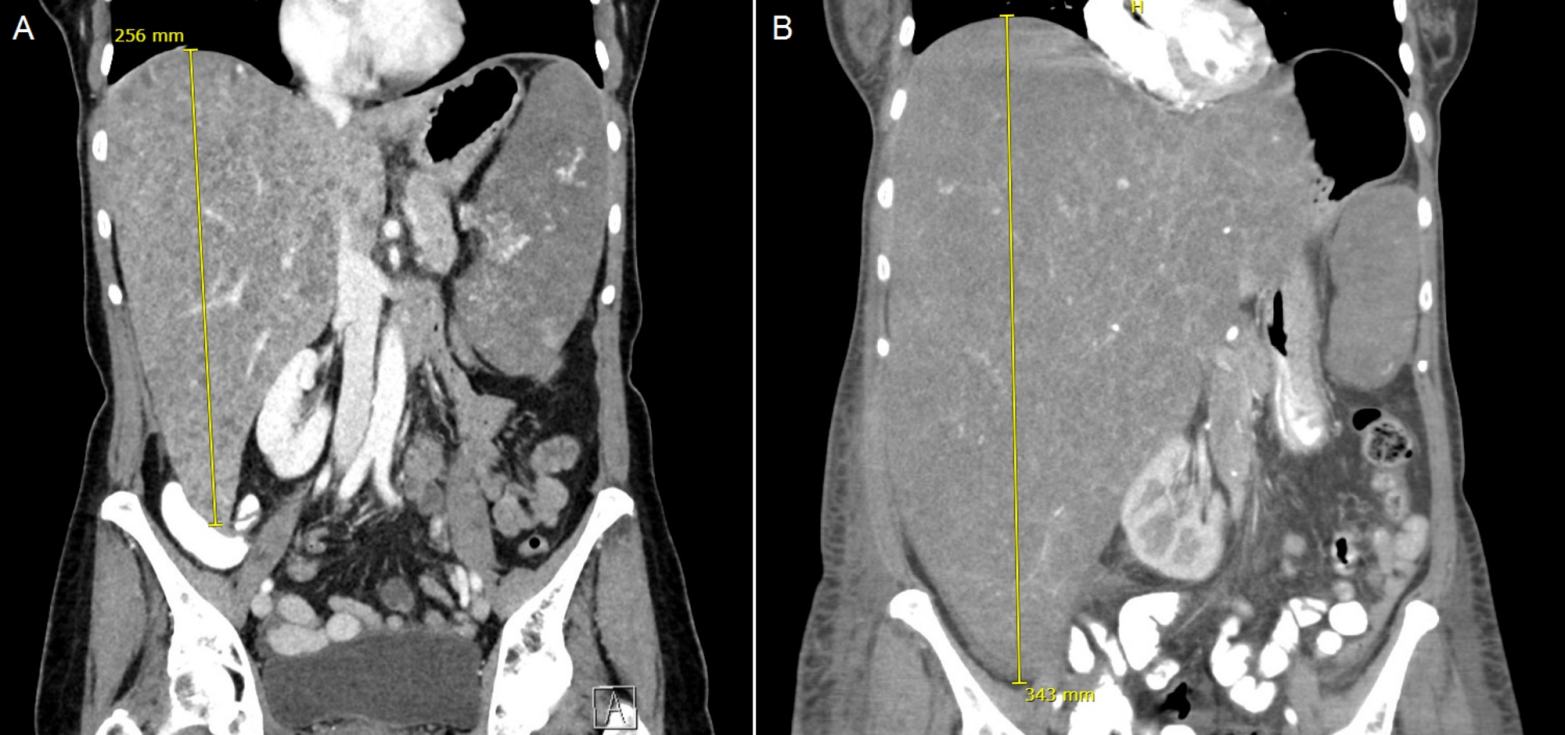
Coronal CT image of the abdomen with massive hepatomegaly. The liver measured 25.6 cm in craniocaudal length on initial imaging (A), and had enlarged to 34.3 cm on subsequent imaging five weeks later (B), displacing the right kidney and with mass effect
